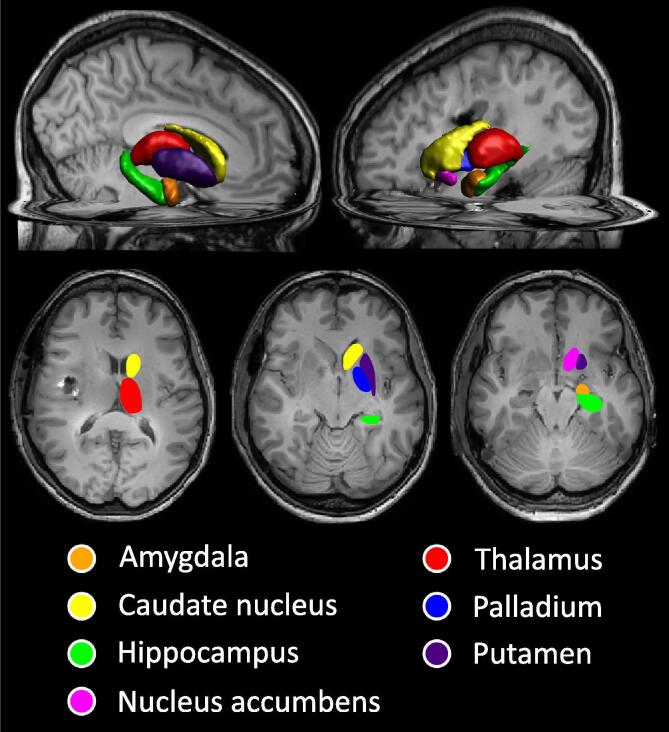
A 3D rendering and axial MR images showing the subcortical grey matter structures being analysed.
